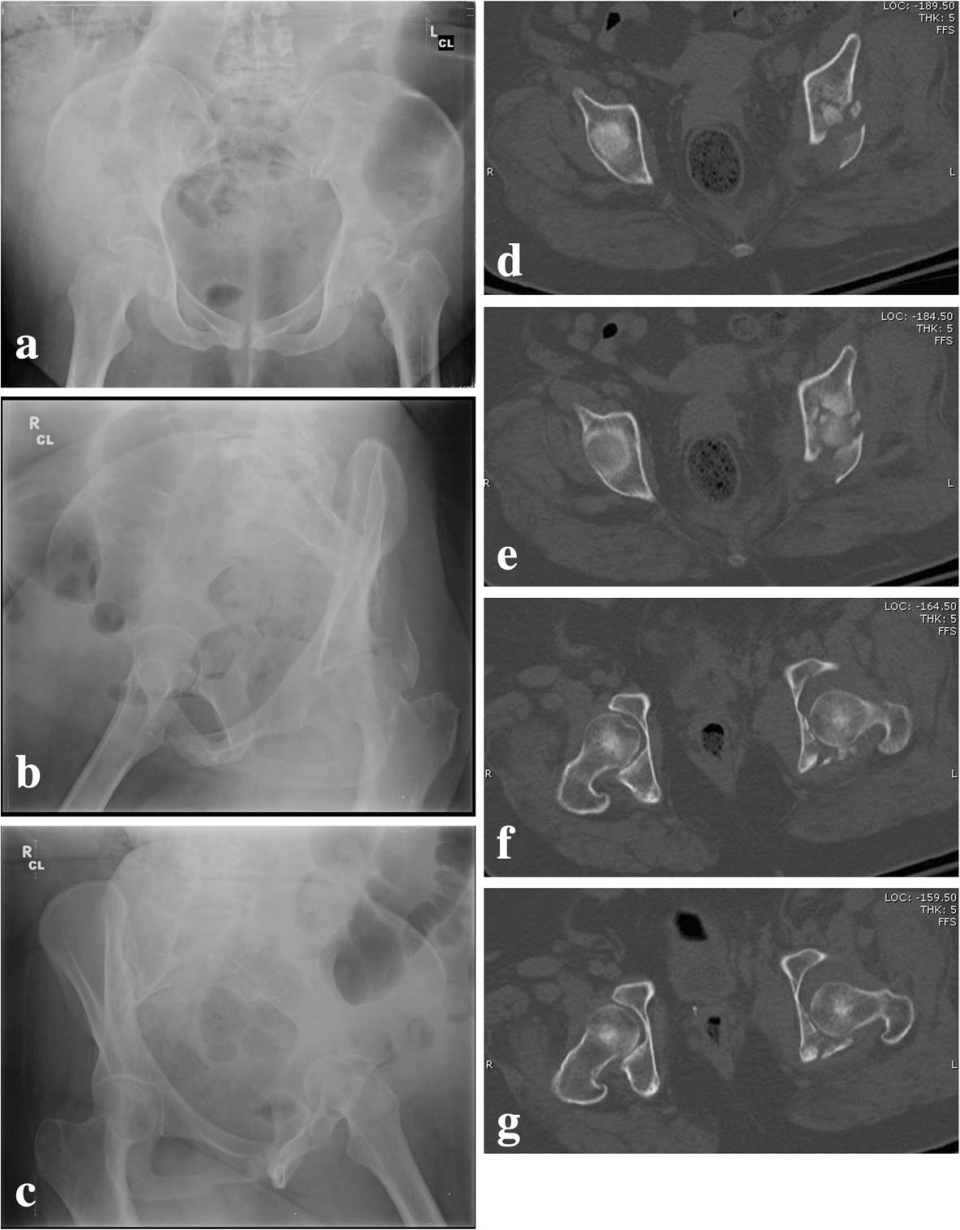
Pre-operative (a–c) radiographs and CT (d–g) of a patient who sustained a comminuted transtectal transverse acetabular fracture with involvement of the posterior wall
